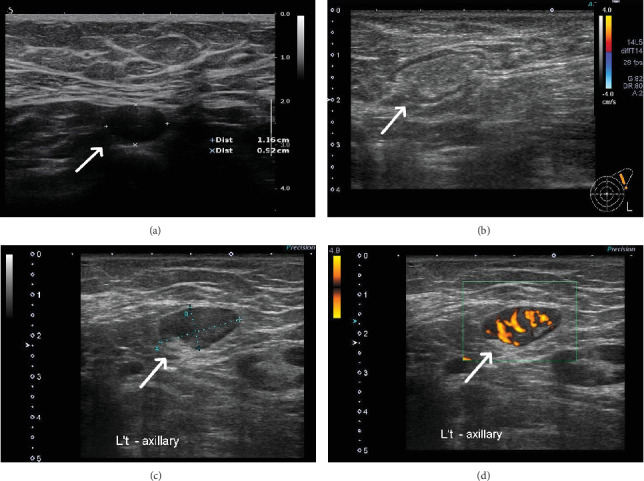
Example for vaccine-related lymphadenopathy. (a) A 50-year-old woman with vaccine-related lymphadenopathy presented as left axillary Level I lymph node with thickened cortex and loss of the normal fatty hilum. (b) Normal lymph node. (c) A 42-year-old woman with vaccine-related lymphadenopathy presented as left axillary Level I lymph node with thickened cortex. (d) Colored Doppler, hypervascularity was presented.
